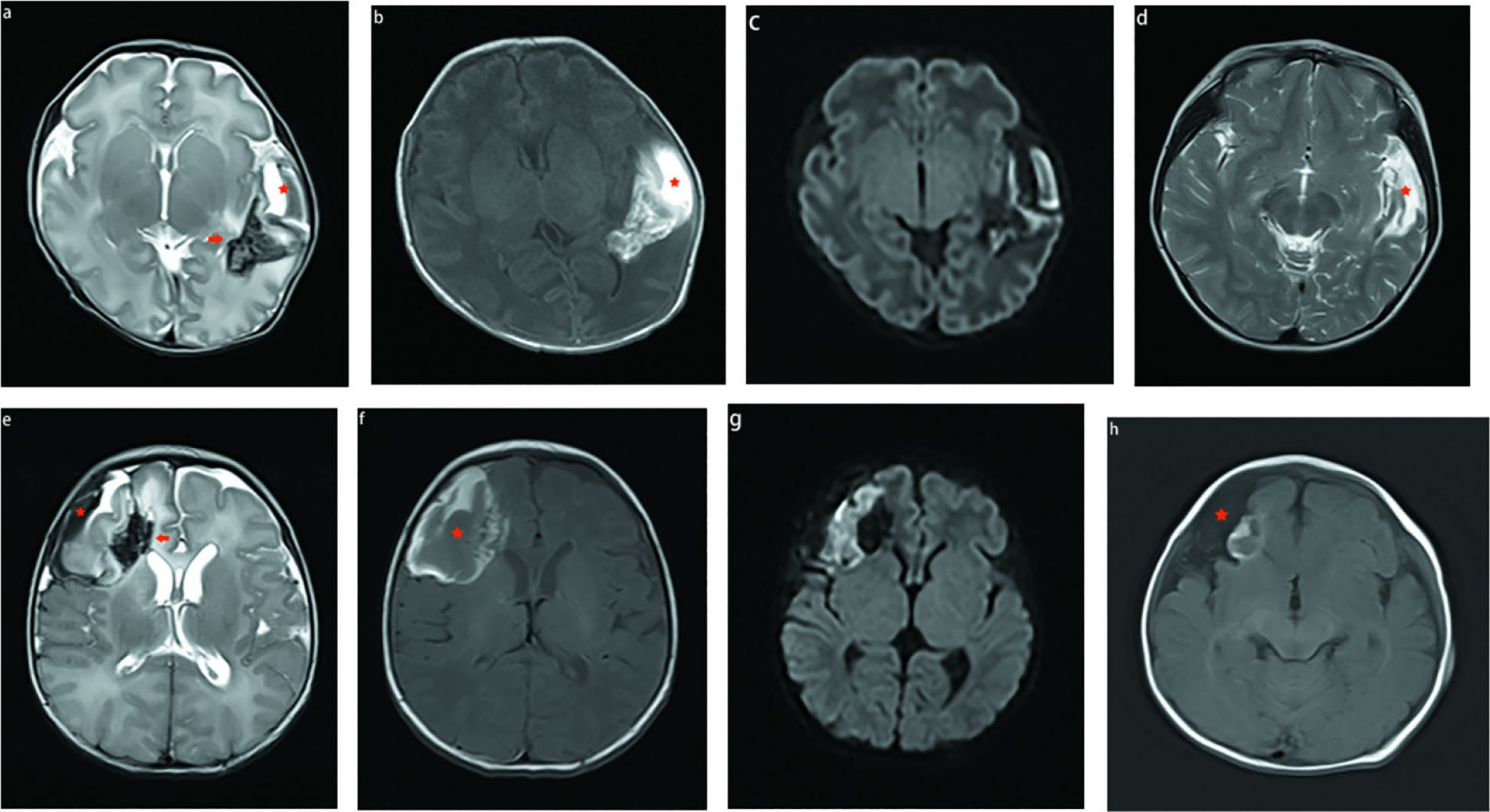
MR images of 2 neonates. The first patient (a–d) is a preterm neonate, and the second patient (e–h) is a term neonate. The first MRI shows (a–c and e–g) a subpial bleed (star), underlying the cerebral cortex and white matter with fan-shaped hemorrhage (arrow) created a sandwich sign. We found the medullary veins were notably enlarged (arrow in a and e). Reexamination after 3 years (d) and 5 months (h) showed that the subpleural hemorrhage and cerebral parenchymal hemorrhage had been absorbed and eventually encephalomalacia and gliosis. Subpial cystic cavities were formed (red star in d and h).
